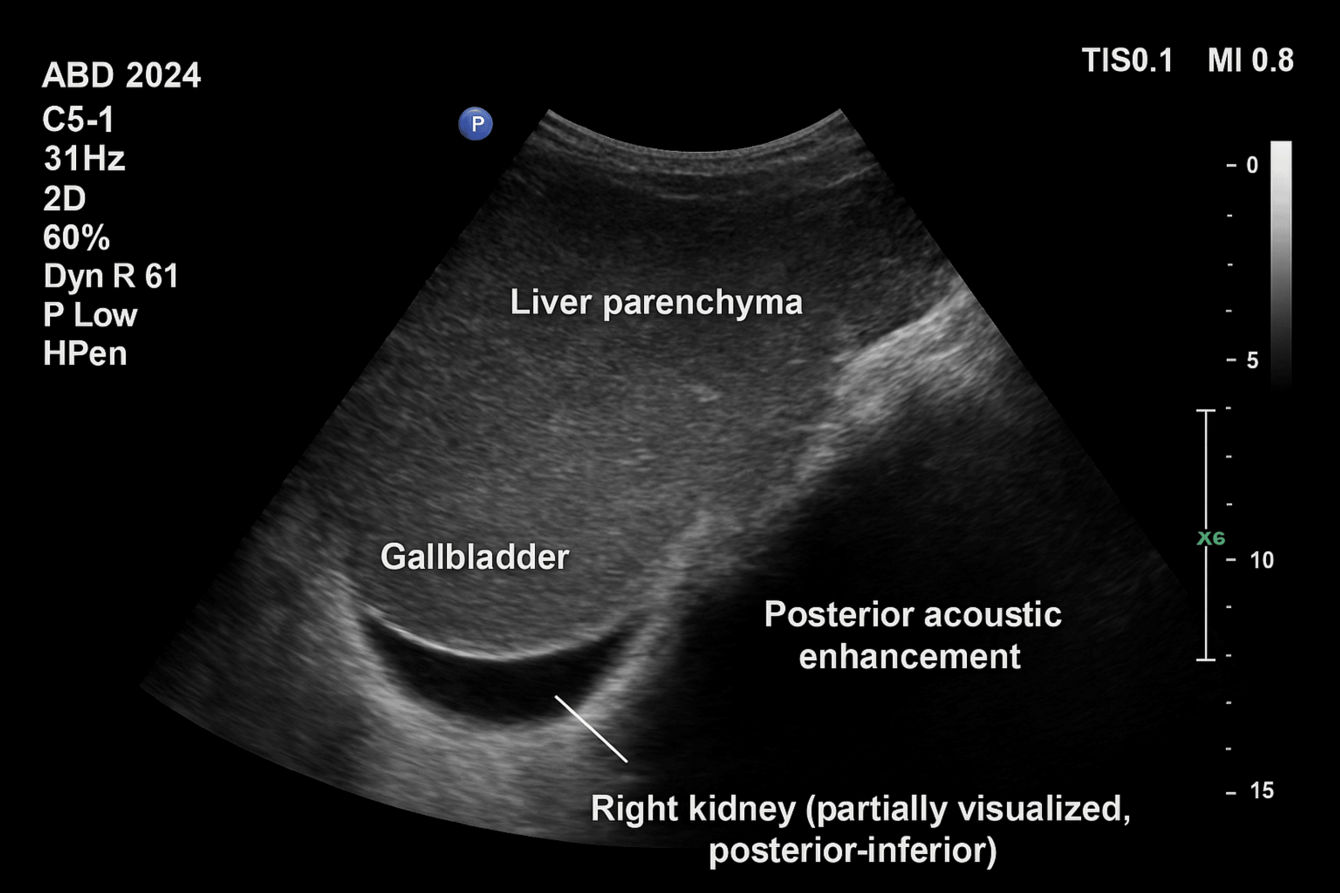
Ultrasonography of case 2
